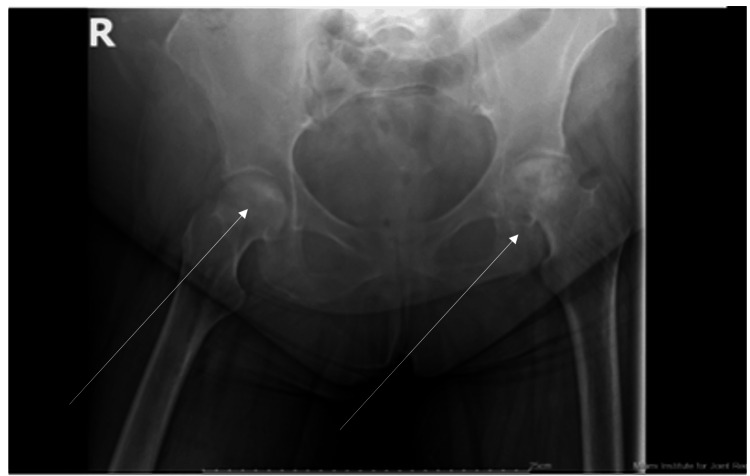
Anteroposterior radiograph of the pelvis. Arrows indicate the area of possible avascular necrosis.
